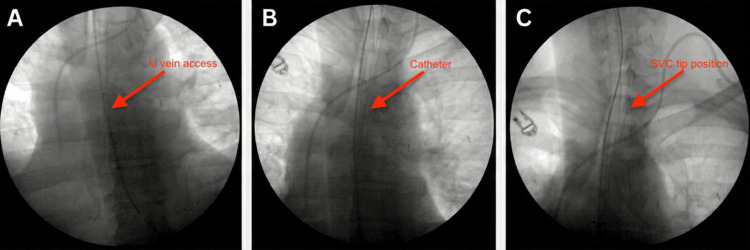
X-ray fluoroscopy. Intraoperative fluoroscopic images obtained during placement of a permanent venous access port. The left panel demonstrates internal jugular (IJ) vein access (red arrow). The middle panel demonstrates the catheter coursing through the central venous system (red arrow). The right panel demonstrates the catheter tip positioned in the superior vena cava (SVC), confirming appropriate placement for systemic therapy (red arrow).
